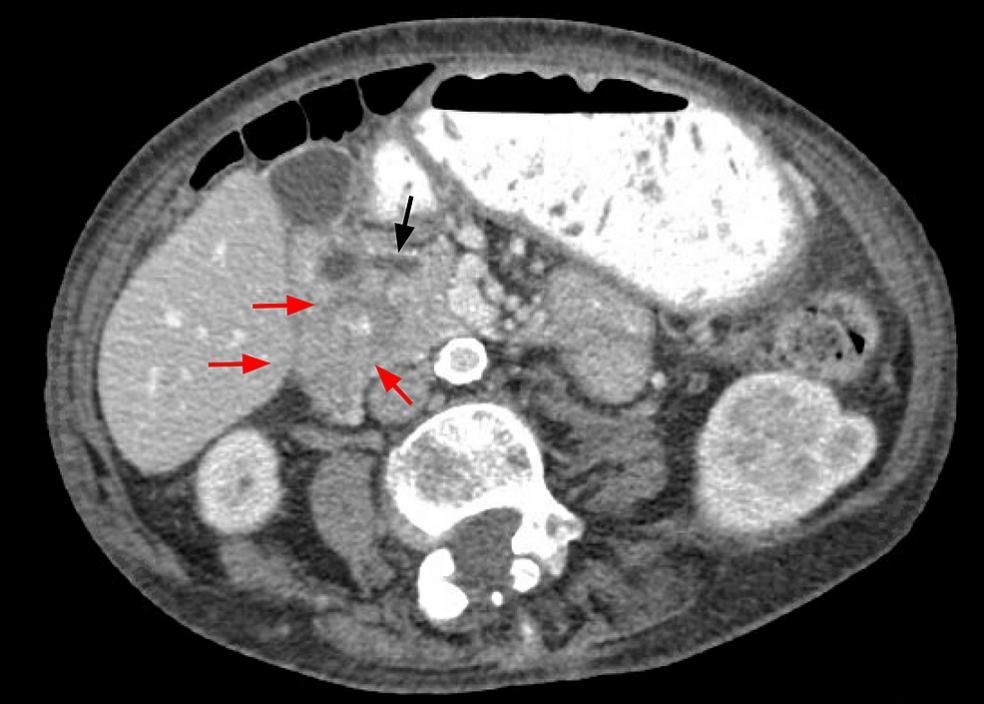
CT abdomen and pelvis with IV contrast in axial view. Shows the dilated pancreatic duct 5 mm (black arrow) and pancreas divisum emptying into a duodenal diverticulum. Intraluminal duodenal diverticulum (red arrows).
